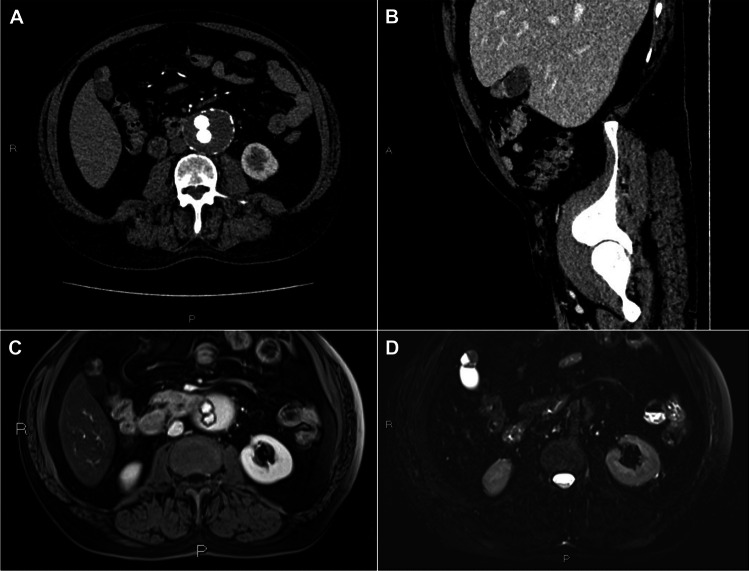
AM-ICN: case #2 imaging features. A CT scan shows arterial enhancement of solid components within mural cystic spaces of the gallbladder fundus (axial plane, A), without washout in the venous phase (sagittal plane, B), confirmed in the portal phase of the MRI (C). In the axial fat-suppressed T2-weighted image, the solid tissue is markedly hypo-intense compared to the adjacent fluid component (D). These imaging findings are suspicious for malignancy
